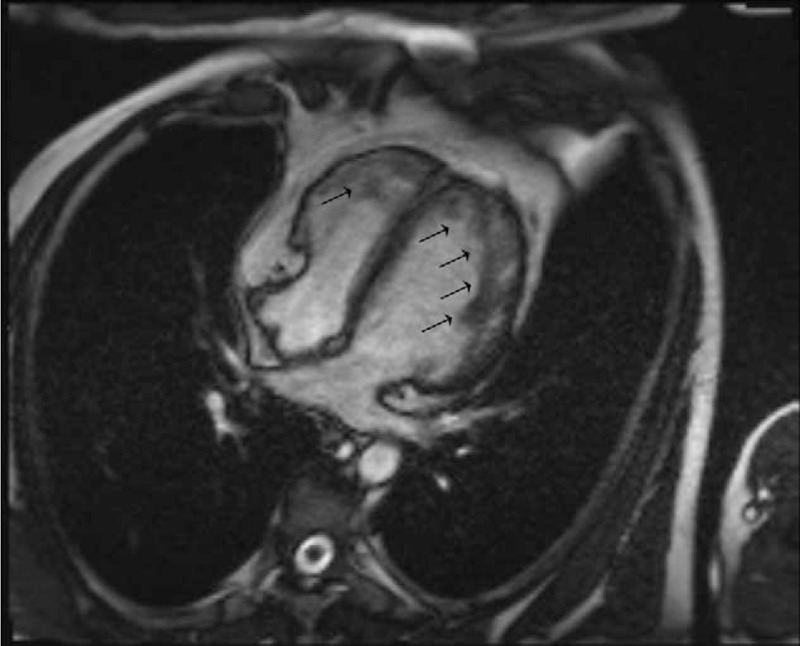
Cardiac magnetic resonance image showing evidence of ventricular non-compaction. Arrows indicate the non-compacted ventricle. The ratio between non-compaction to compaction of the ventricular myocardium is >2.3 at the end of diastole, which is one of the diagnostic criteria for LVNC.^[15]^
